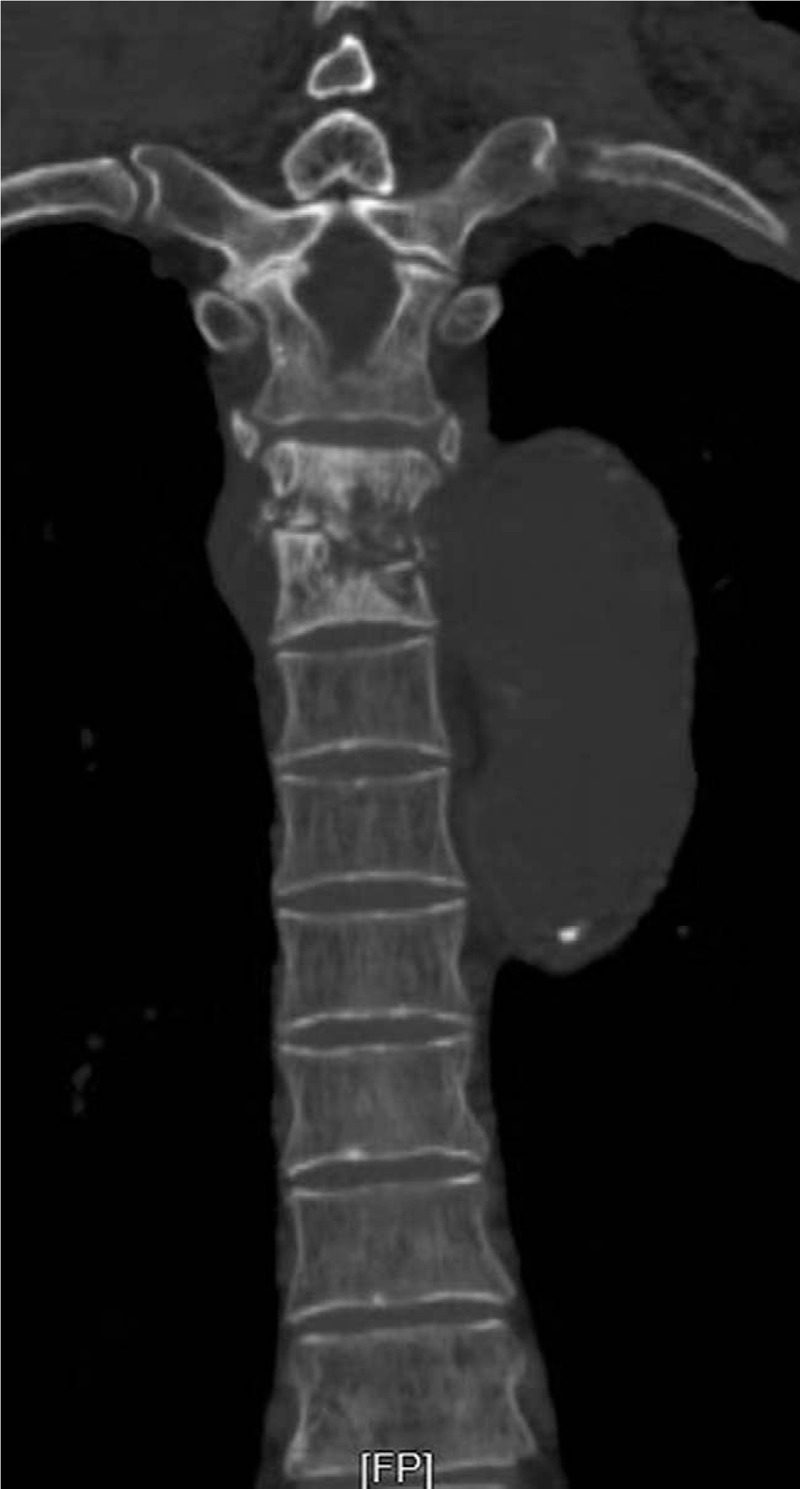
Chest CT showing osteolytic destruction at the 5-6th bodies of thoracic vertebrae and the saccular pseudoaneurysm in descending thoracic aorta. CT = computed tomography.
